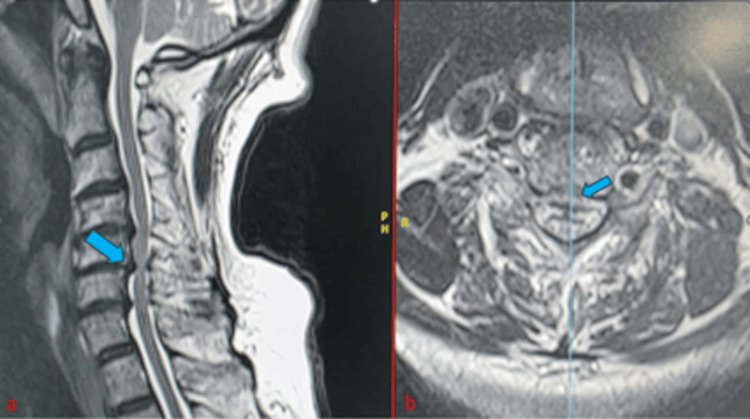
Magnetic resonance imaging scans presenting the preoperative radiological assessment for patients who manifest cervical disc disease signs and symptoms (a) Sagittal and (b) axial. The blue arrow indicates the most prominent degenerative disc herniation.
